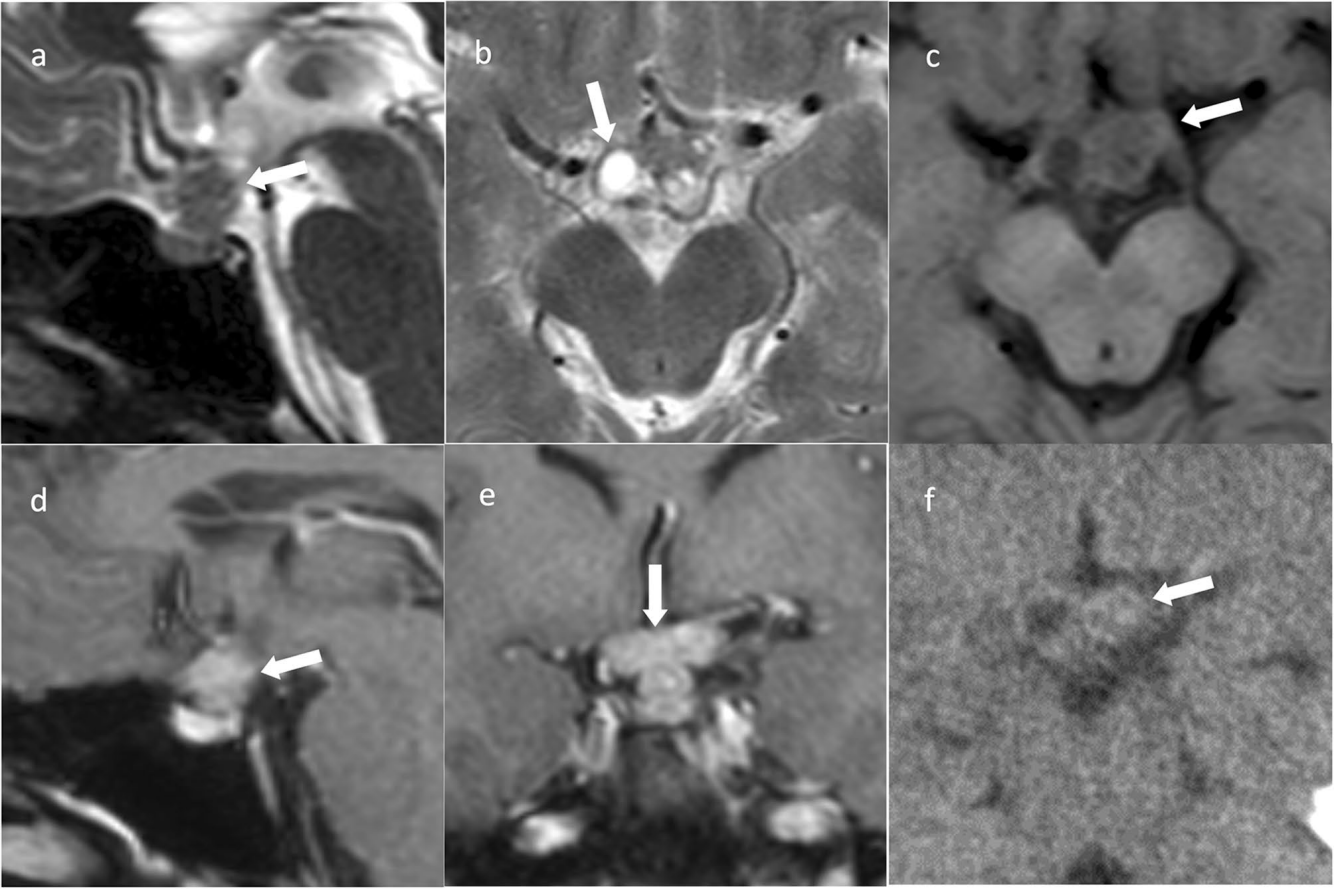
Germinoma. An approximately 30-year-old male with poor vision and no uropathy at the time of magnetic resonance imaging. a Sagittal T2WI shows a mass in the upper part of the sella turcica with isointensity compared to gray matter (arrow). b Axial T2WI shows a cystic component (arrow). c Axial T1WI shows an isointensity mass (arrow). d Sagittal contrast-enhanced T1WI shows relatively uniform contrast enhancement (arrow). e Coronal contrast-enhanced T1WI shows infiltration of the optic chiasm (arrow). f Non-contrast computed tomography shows isodensity (arrow). Germinoma was diagnosed based on craniotomy biopsy. Post-biopsy, steroids were administered, and central diabetes insipidus/arginine vasopressin deficiency (CDI/AVP-D) developed. This was attributed to the presence of an adrenocorticotropic hormone secretory defect, a phenomenon known as masked CDI/AVP-D
